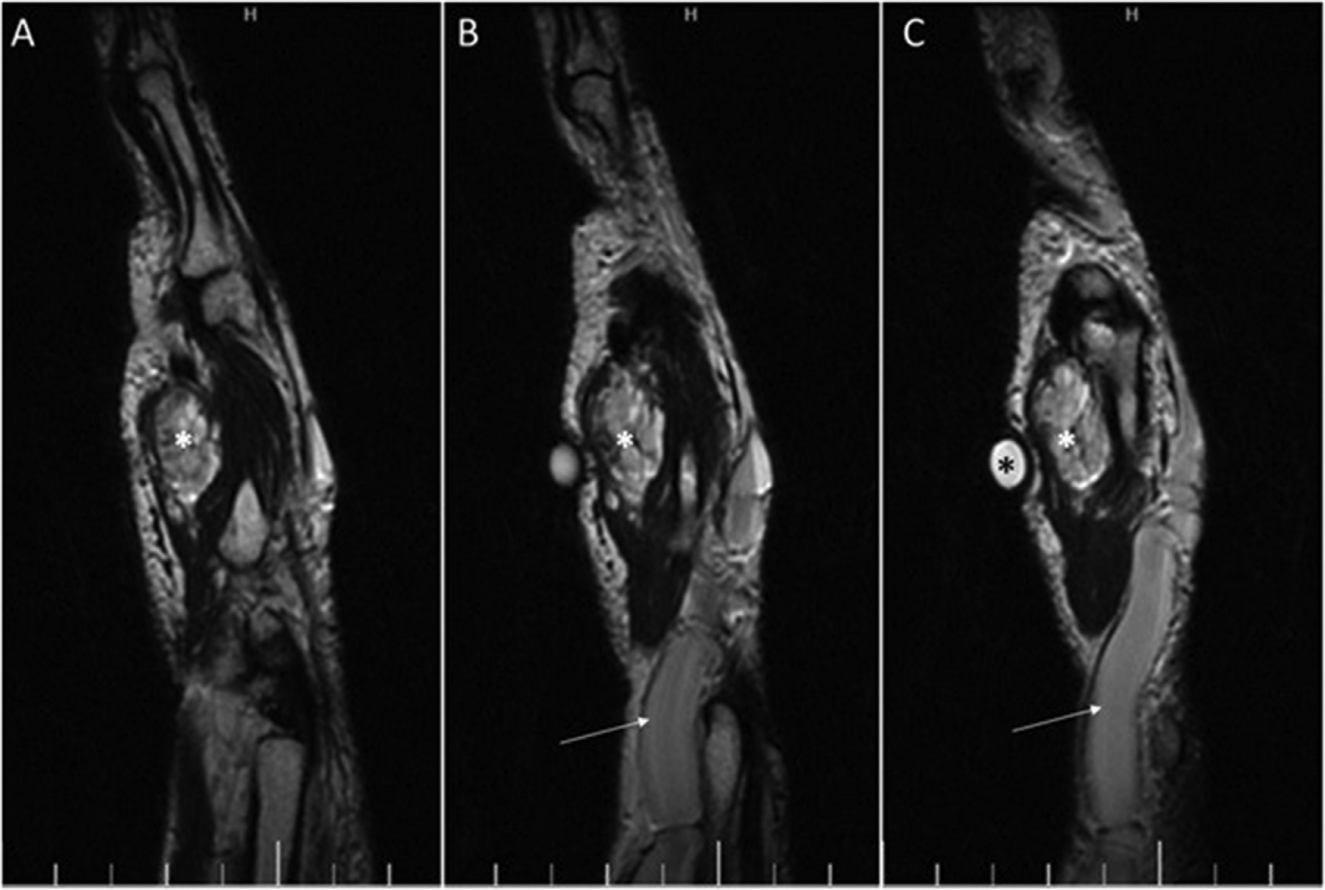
Sagittal T2 weighted MRI of wrist and hand. (A-C) Hyper signal soft tissue mass (white asterisk) is seen in the palmar aspect of the hand at the site of lump palpation (black asterisk indicates the body marker). The aneurysmal dilatation of basilic vein is noted (arrow).
